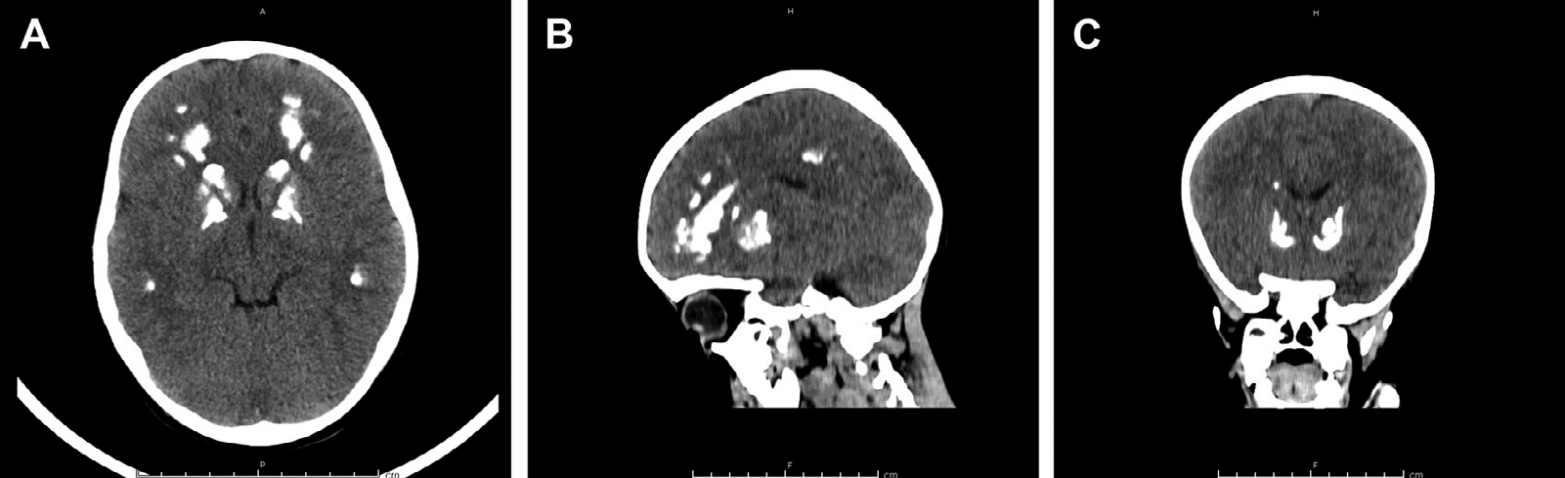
Brain computed tomography (CT) scan showed abnormal symmetrical calcifications seen in the both basal ganglia, thalami and subcortical white matter (A-C) associated with increased bone density of the skull and upper cervical spine (B and C).
